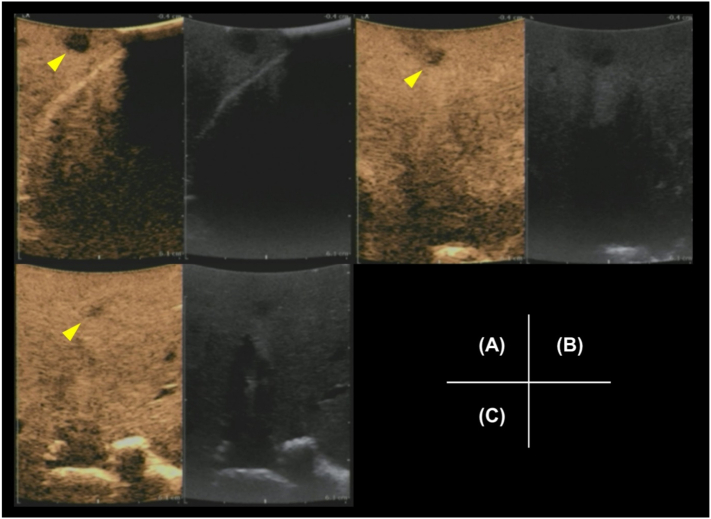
Intraoperative sonazoid-enhanced ultrasonography of the hepatic lesions. Lesions (yellow arrowheads) are noted in segments 3–1 (A), 5 (B), and 8 (C). No lesions are observed in segments 3–2 and 4. (For interpretation of the references to colour in this figure legend, the reader is referred to the web version of this article.)
